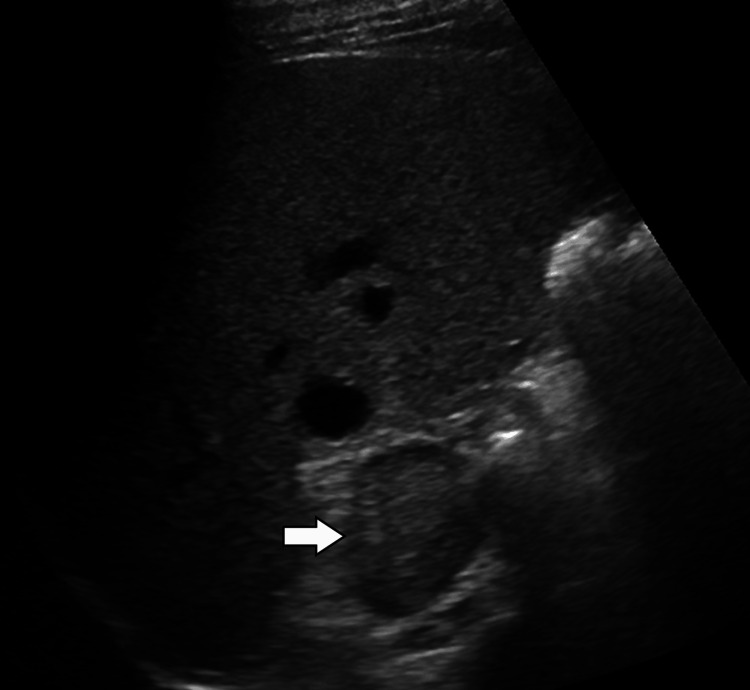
Ultrasound of the right adrenal gland Greyscale ultrasound image of the right adrenal region demonstrates a well-defined, hypoechoic soft tissue mass (arrow). No obvious cystic or calcific components are seen.
